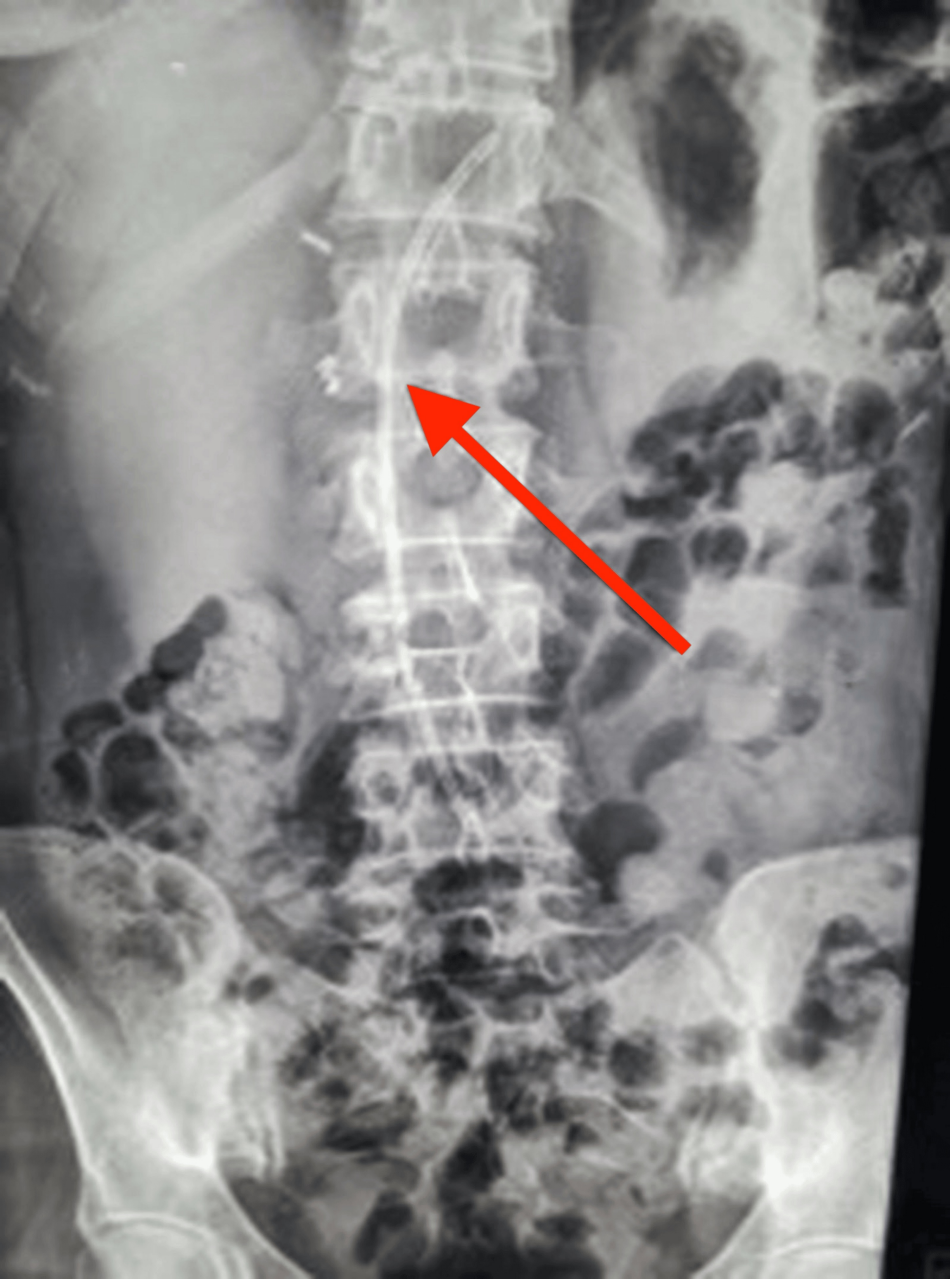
Abdominal plain radiography after the first ERCP/SpyGlass™. A plastic stent (arrow) placed in the first ERCP, post-cholecystectomy clips, and an unidentified tubular radiopaque object are observed ERCP: endoscopic retrograde cholangiopancreatography
